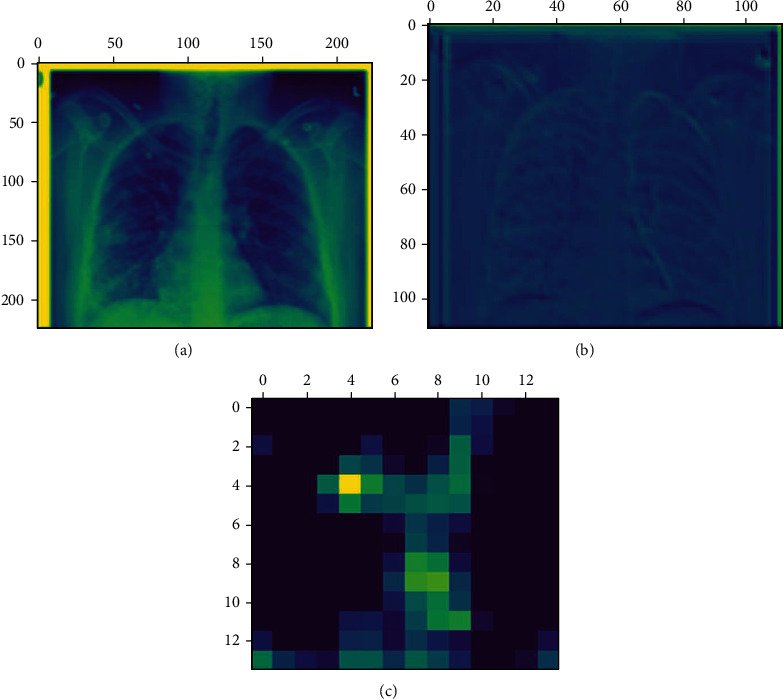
The dimension reduction technique as visualized in VGG16 networks: (a) the 24^th^ channel of the activation for the 1^st^ layer of the COVID-19 X-ray image, (b) the 1^st^ channel of the activation for the 5^th^ layer of the COVID-19 X-ray image, and (c) the 1^st^ channel of the activation for the 17^th^ layer of the COVID-19 X-ray image.
